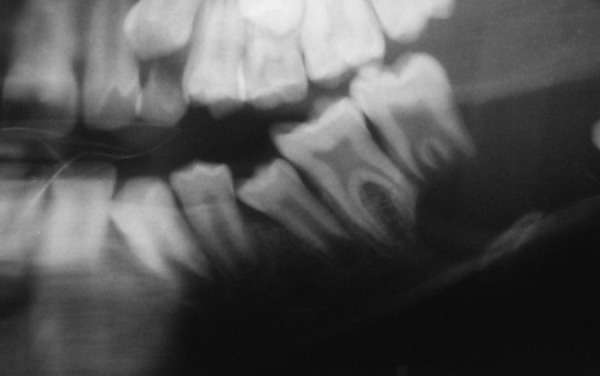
Radiograph showing radiolucency extending till the base of mandible surrounding the crown of the unerupted third molar resembling the dentigerous cyst
